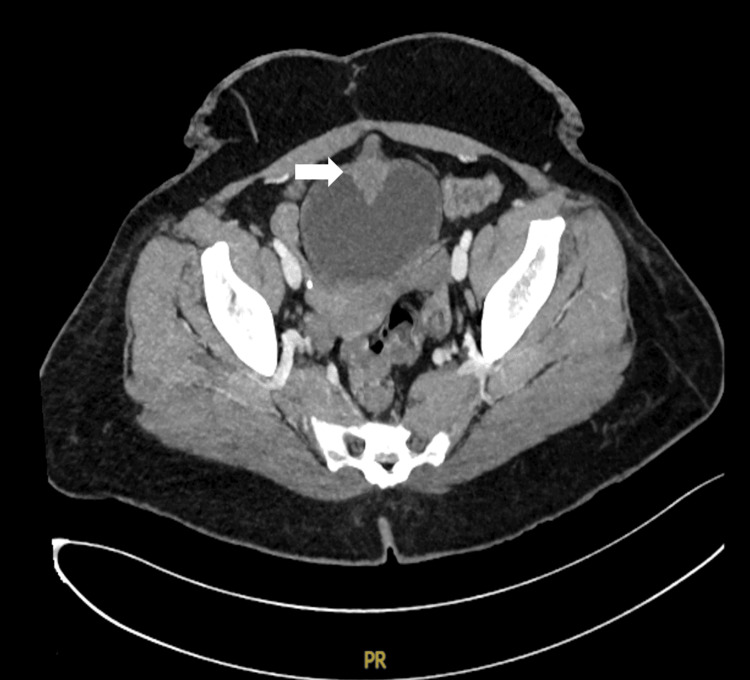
CT axial section demonstrating soft tissue density over the anterior wall of the urinary bladder White arrow pointing to the tumor CT: computed tomography
